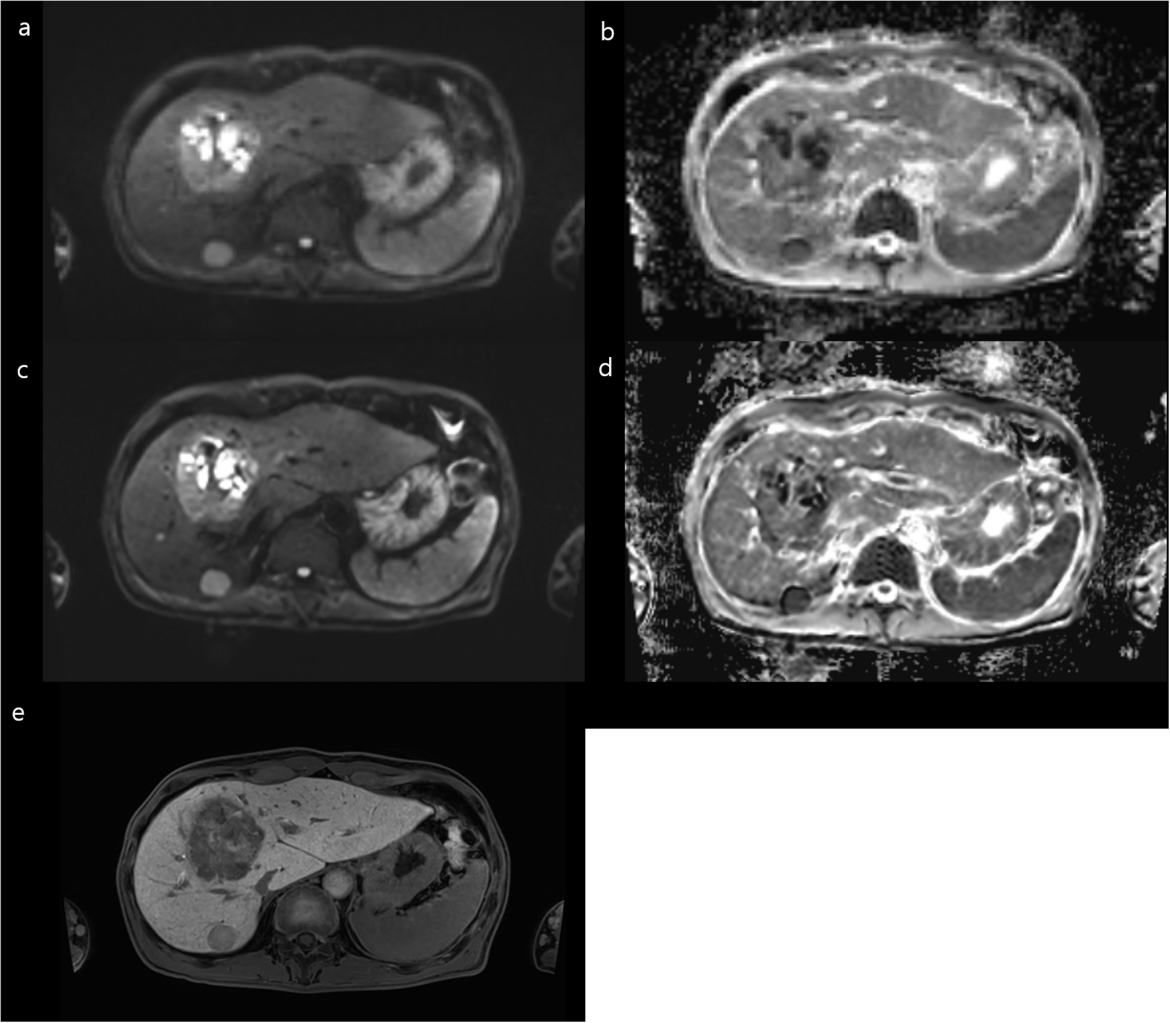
A 6.5-cm hepatocellular carcinoma based on typical imaging features and elevated AFP in segment 8 in a 49-year-old male patient with hepatitis B cirrhosis showing a blurred margin on FB-C-DWI (a) and the ADC map (b), and a clear margin on FB-DL-DWI (c) and the ADC map (d).
